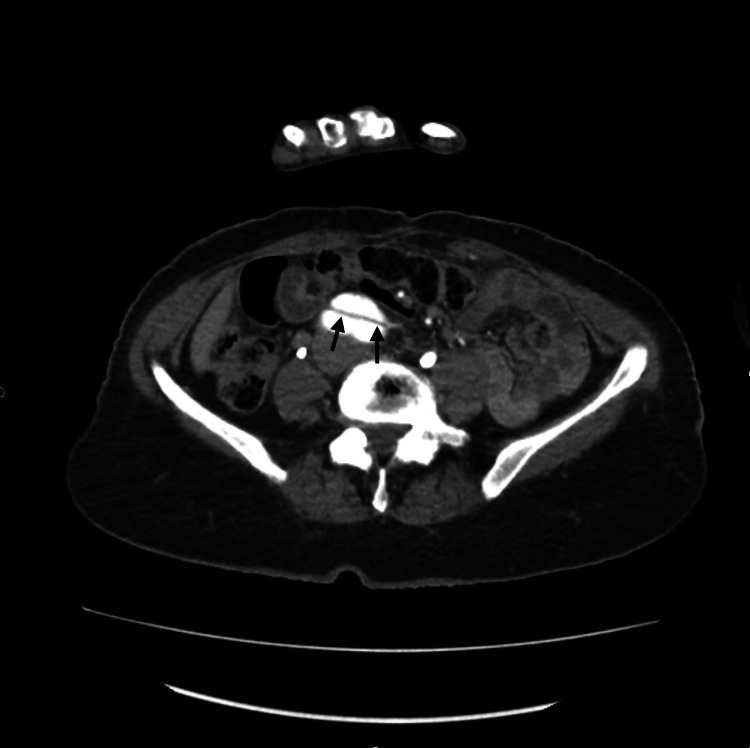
CTA axial image of the middle abdomen with a repaired DeBakey type 1 dissection. Arrows indicate the false lumen of the dissection CTA: computed tomography angiography
